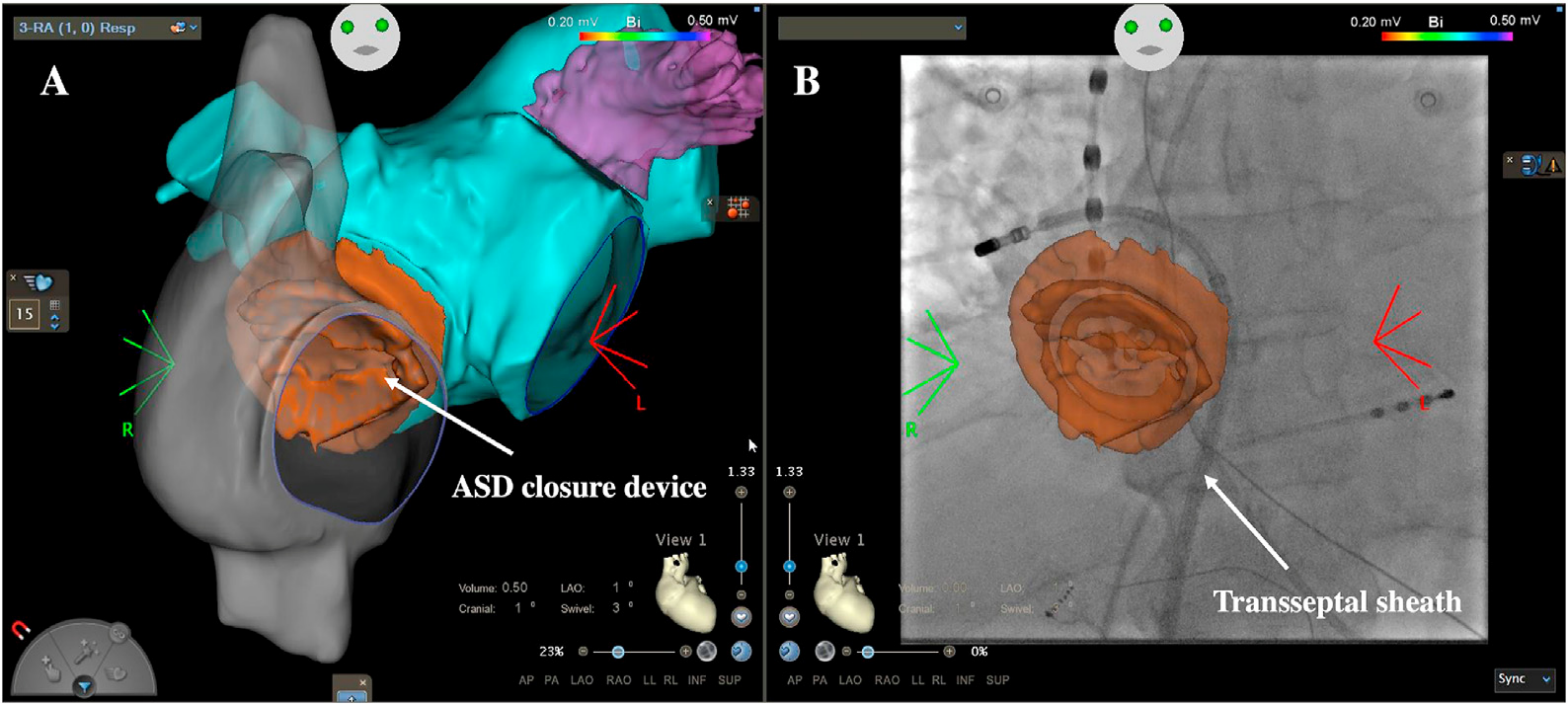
Transseptal access in a patient with atrial septal defect closure device. A: 3D anatomical reconstruction of the right atrium (gray) and merging with the computed tomography (CT) scan (left atrium in blue and ASD closure device in orange) to locate the optimal puncture site (anteroposterior view). B: Fluoroscopy view with superposition of the ASD closure device showing the transseptal sheath inserted just inferiorly to the device. The ablation catheter is placed in the right sided pulmonary vein.
